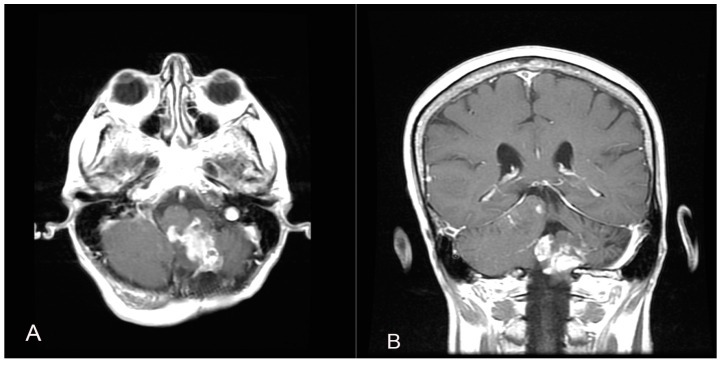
A follow-up contrast-enhanced brain MRI two years after the first surgical intervention shows the presence of a recurrence in the left cerebellar area with inhomogeneous enhancement (A,B).
